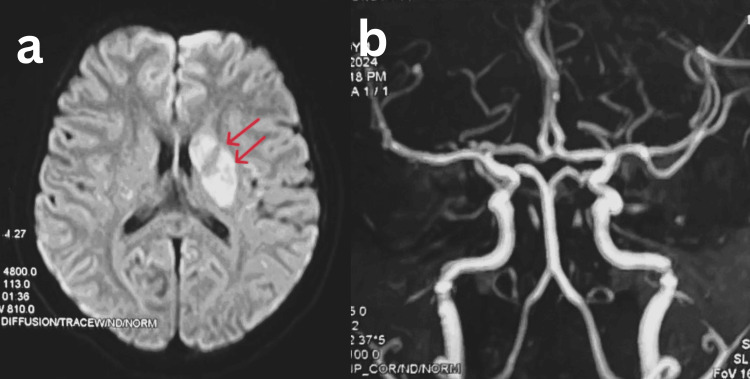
Magnetic resonance imaging with magnetic resonance angiography of the brain. (a) MRI revealed diffusion restriction in the left corona and lentiform region. (b) MRA showed no evidence of large-vessel occlusion.
